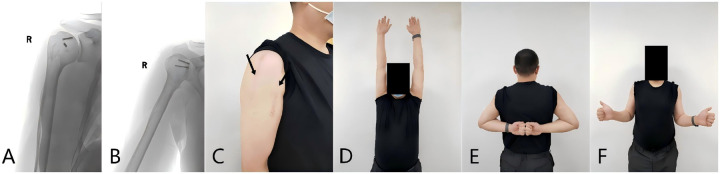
(A,B) Radiographs obtained 5 years after revision surgery show no radiographic signs of humeral head osteonecrosis. (C) The two black arrows indicate the incisions from the two procedures: the deltoid-splitting approach and the deltopectoral approach. (D–F) At 5 years after revision surgery, shoulder function had recovered to an excellent level.
